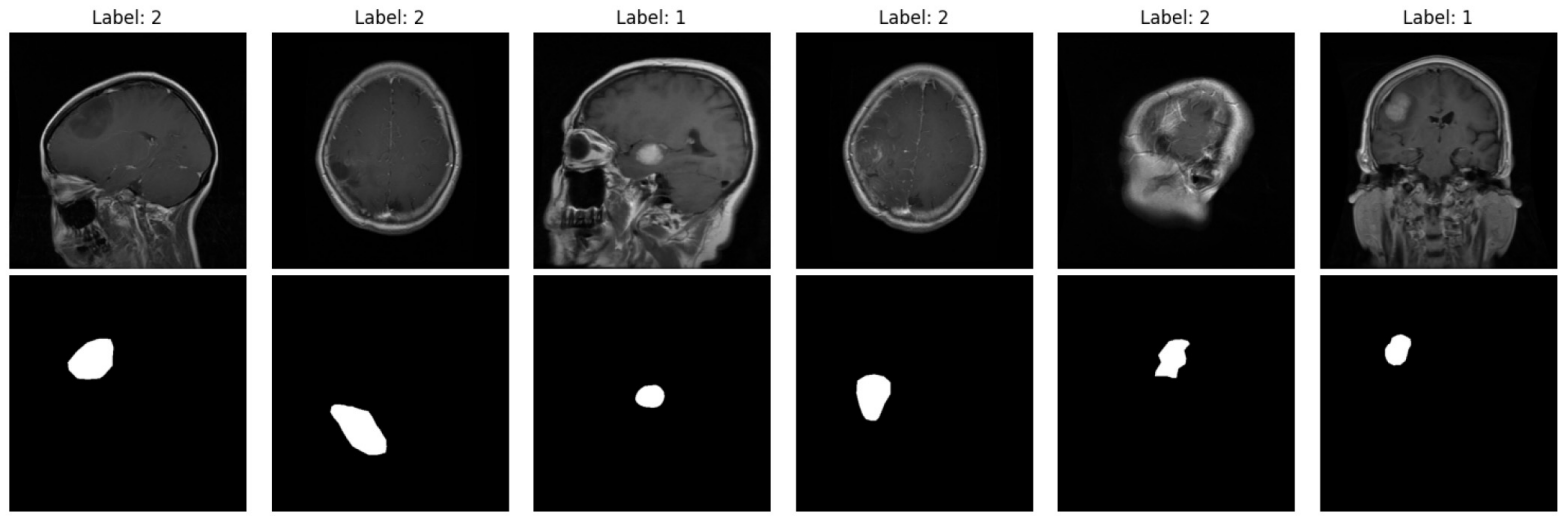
Example MRI slices (top) with corresponding tumor masks (bottom) from the Figshare dataset, used to validate tumor regions before bounding-box annotation.
